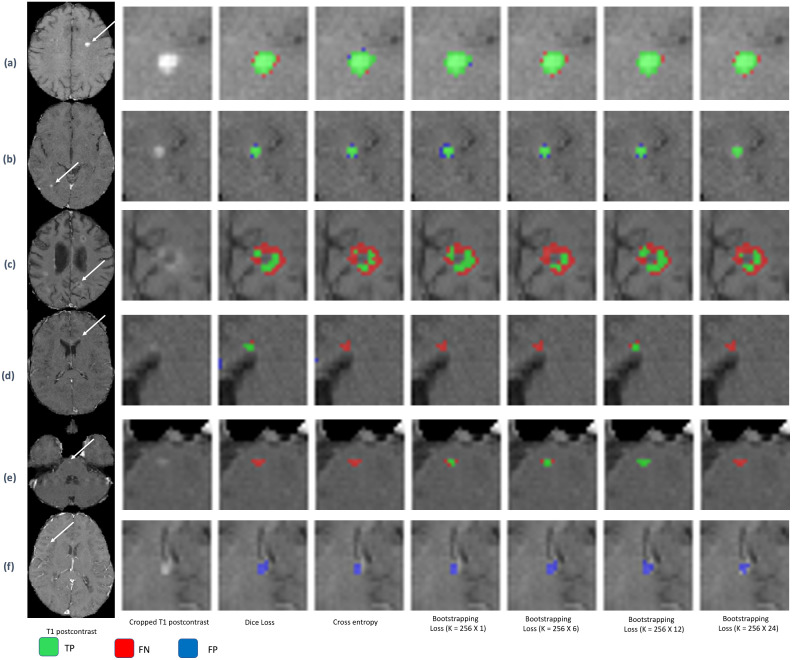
Predicted images for different loss functions. Each row represents image slices from different MRI sequences. The first column represents T1 post-contrast images and the second column represents the enlarged T1 post-contrast images around the lesion of interest. The other columns are performances of UNet and random forest model for different loss functions. Red regions are false negative segmentation, green areas are true positive, and blue regions are false positive regions. The white arrows are used to identify the lesion of interest. The first two rows (a and b) show example of lesions which are identified by all loss functions. The third row (c) shows change of lesion segmentation volume by loss functions. Fourth and fifth rows (d and e) show the improvement of segmentation due to bootstrapping loss function. The last row (f) shows example of false detection.
